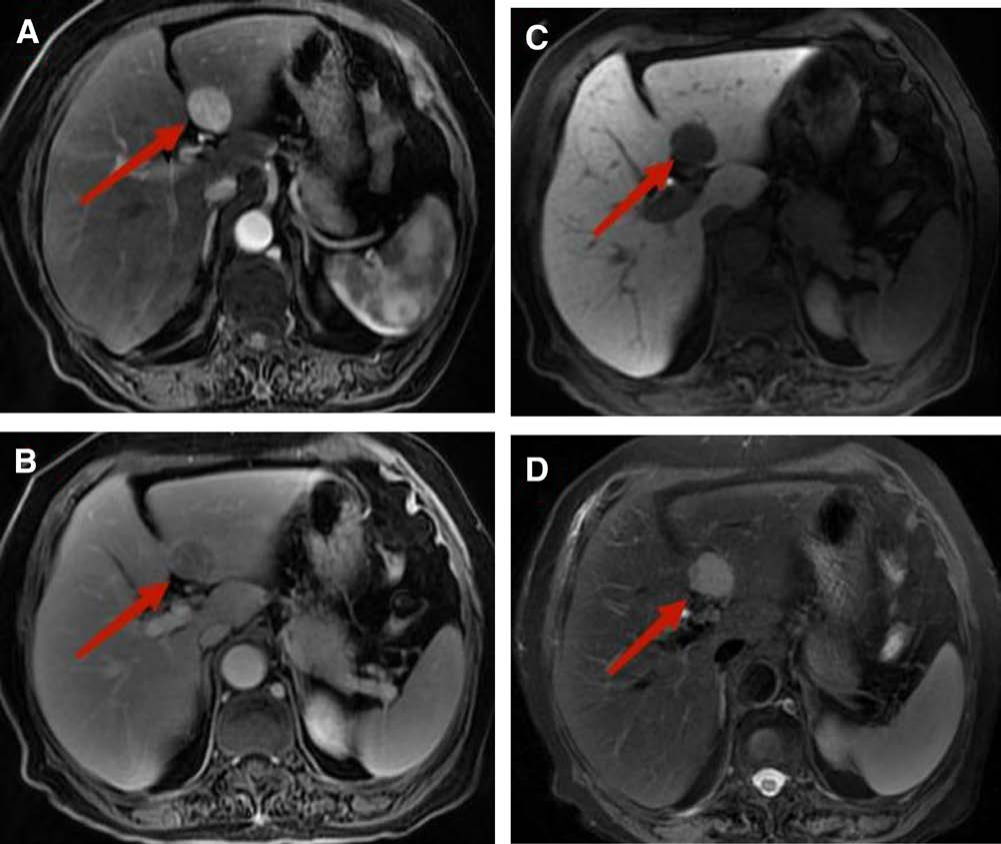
(A) Liver MRI with disodium gadolinite showing significant enhancement during the arterial phase; (B) Lesion signal is lower than liver signal during the venous phase; (C) Low signal during the excretory phase; (D) Long signal decay during the T2 phase.
